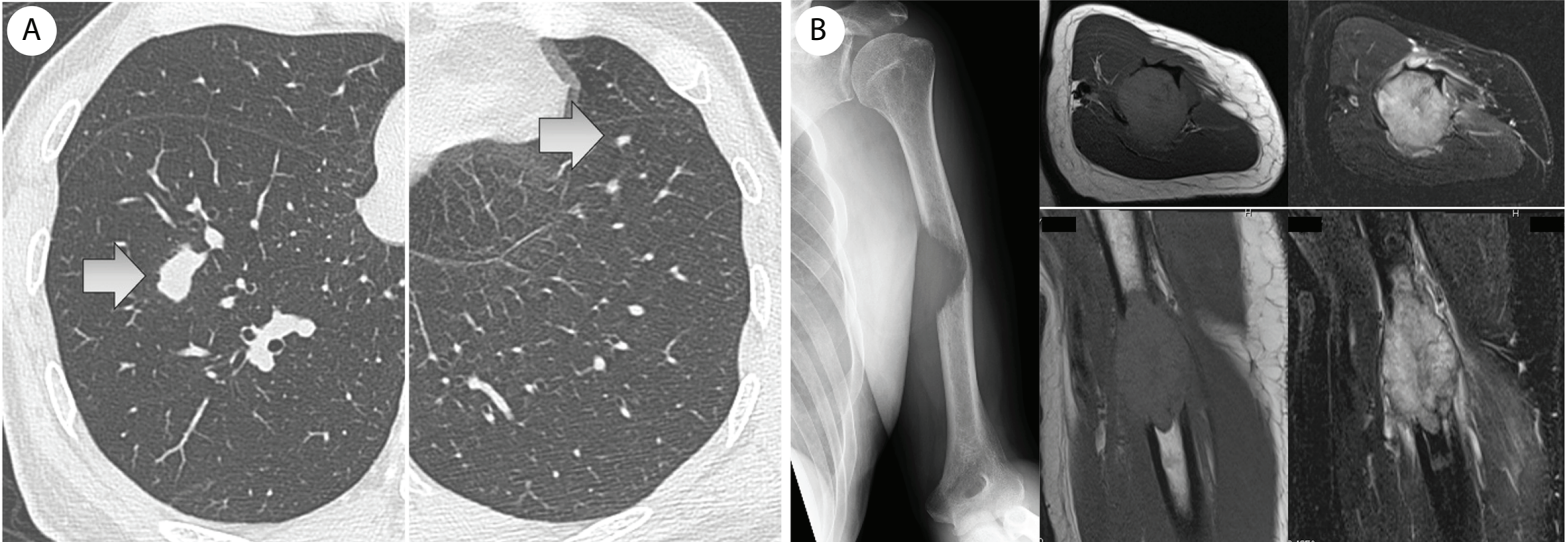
(A) One year after the left nephrectomy, two lung metastases were revealed by computed tomography. (B) Eight months later, a left humerus fracture with osteolytic change was revealed by X-ray. Magnetic resonance imaging (MRI) showed an extraskeletal mass at the fracture site (left: T1-weighted image, right: fat-suppressed T2-weighted image), and it was considered to be a pathological fracture due to bone metastasis.
